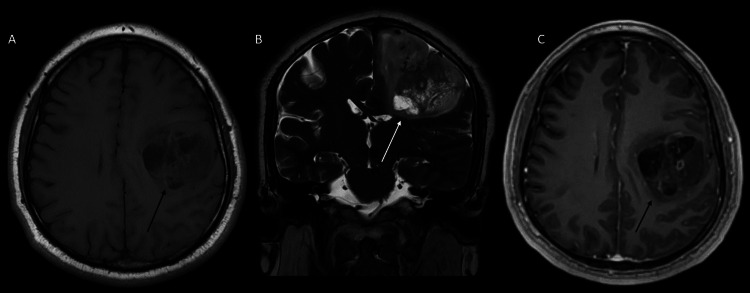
Brain MRI (April 2022). (A) T1-weighted, axial section; (B) T2-weighted, coronal section; (C) contrasted BRAVO, axial section. Left frontoparietal intra-axial lesion, solid heterogeneous with hyperintense areas in T1, is surrounded by slight perilesional edema, affecting the superior frontal gyrus, middle frontal gyrus, pre-postcentral gyrus, conditions mass effect with caudal compression of the adjacent convolutions, as well as the frontal recess and medialization of the uncus. Intravenous contrast shows heterogeneous and irregular enhancement. Compared to the previous study, it presents greater extension and mass effect. MRI: magnetic resonance imaging
